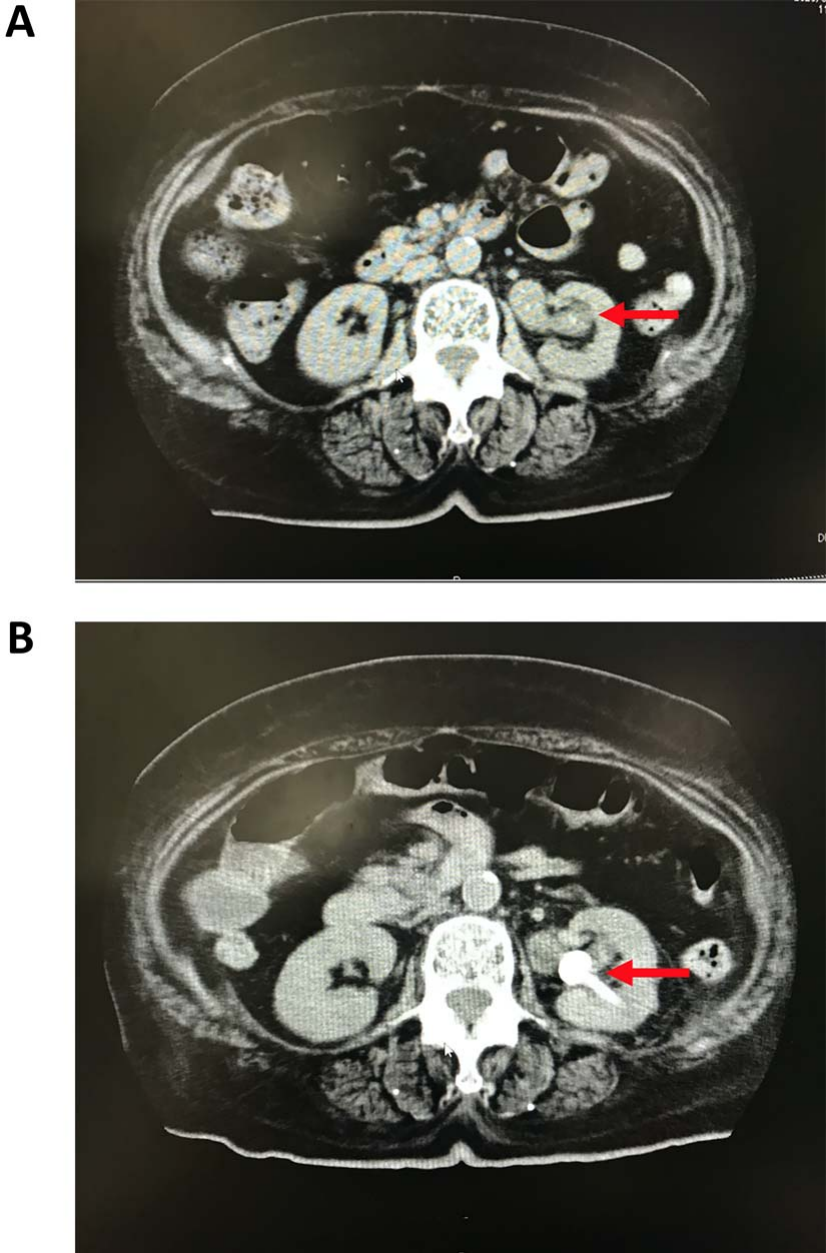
Computed tomography image of percutaneous nephrostomy. (a) Debris present in the left dilated renal pelvis (arrow). (b) The percutaneous nephrostomy tube properly placed in the left kidney (arrow).
